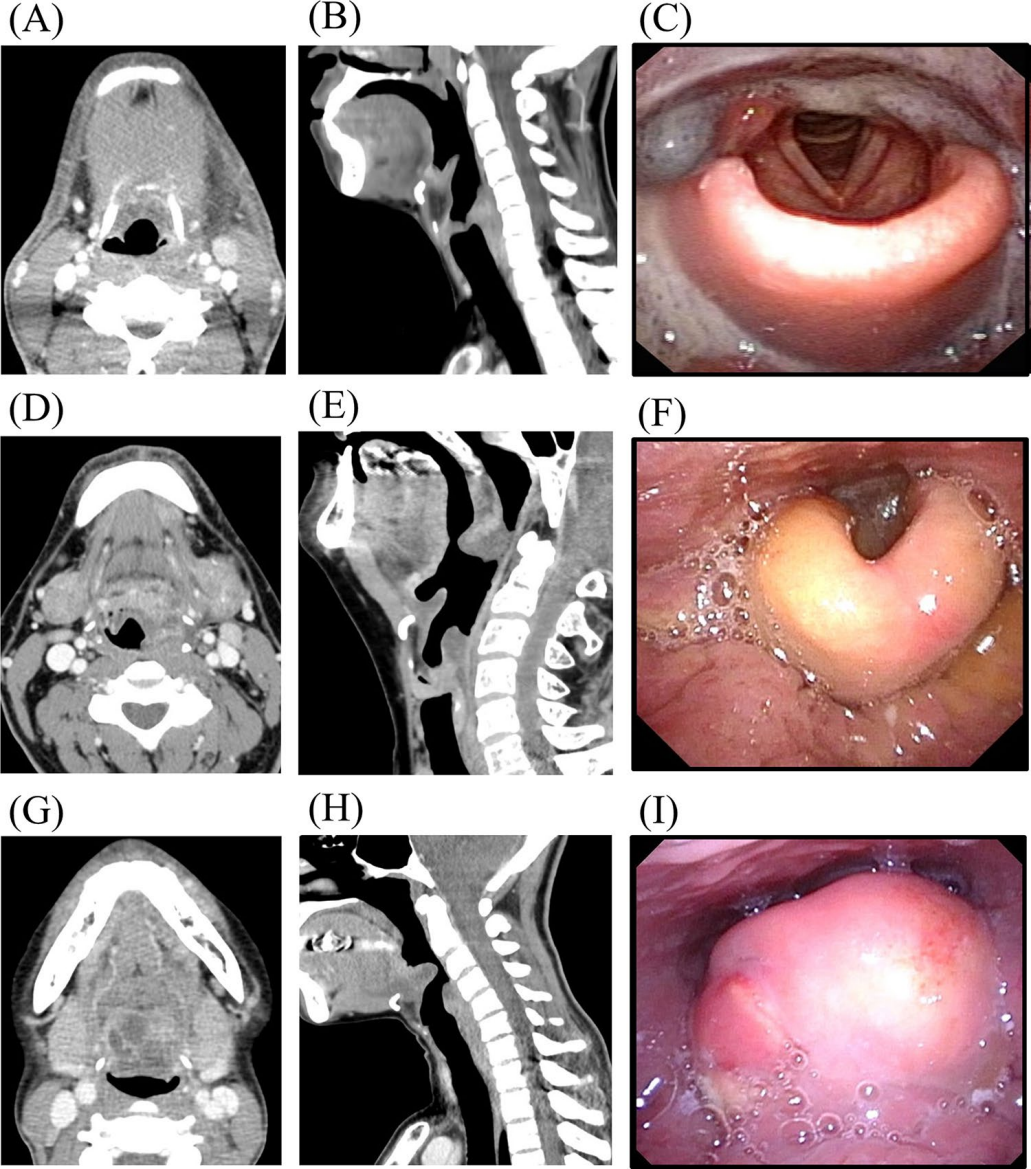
Grading of laryngeal edema (arytenoid edema). Grade I: slight swelling on axial CT image (A), sagittal CT image (B), and laryngoscopy image (C). Grade II: moderate swelling on axial CT image (D), sagittal CT image (E), and laryngoscopy image (F). Grade III: severe swelling on axial CT image (G), sagittal CT image (H), and laryngoscopy image (I)
